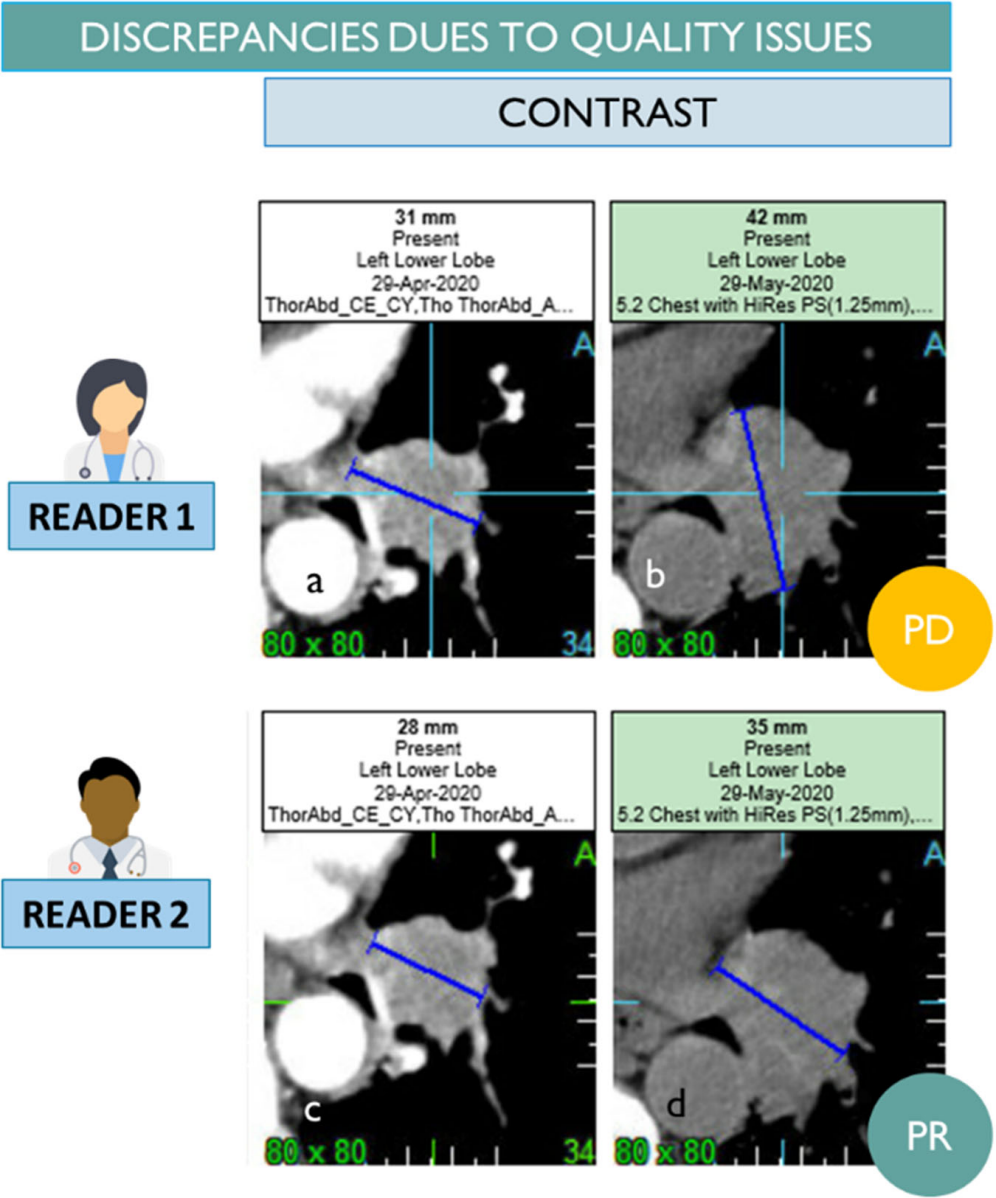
Image quality-related discrepancy. One lung para-mediastinal target is measured with mediastinal windowing and good visualization of adjacent vascular structures (a, c). During the follow-up, one CT evaluation is performed without contrast injection (intercurrent contra-indication). The higher inter-reader variability of measurement due to the lack of contrast in the image resulted in a progression discrepancy (b, d)
